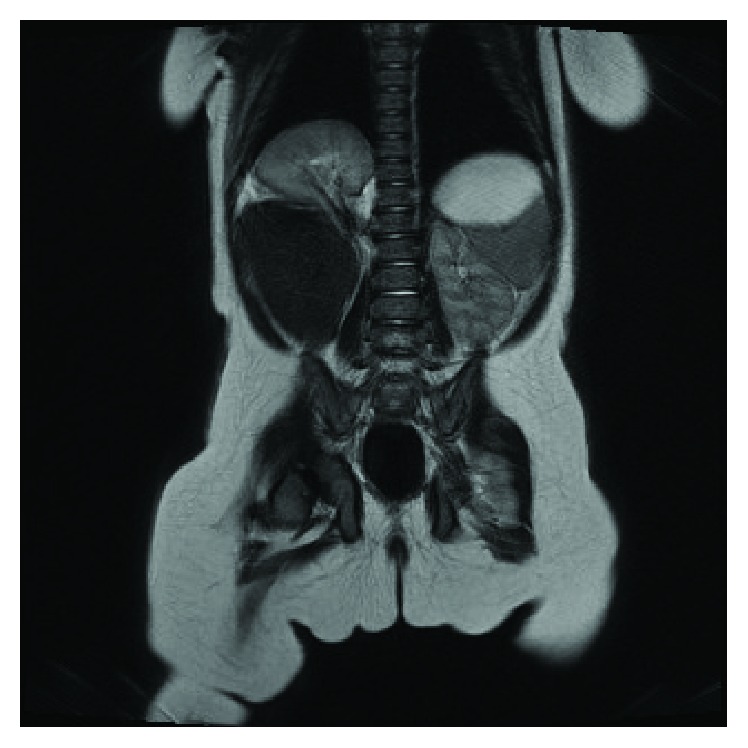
MRI coronal view of the abdomen and the chest. The right kidney appears in a subdiaphragmatic position.
